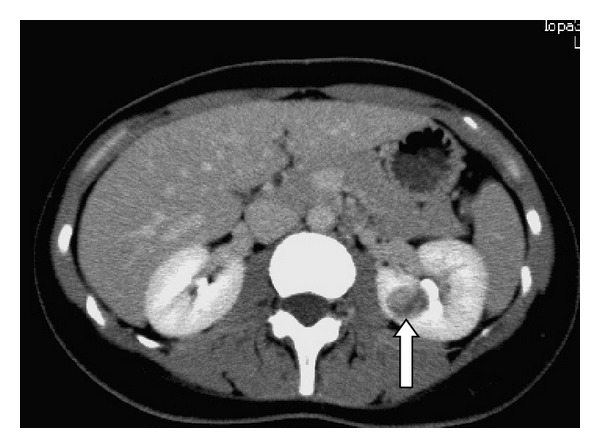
Enhanced CT showing the tumor (excretory phase) (arrow).
